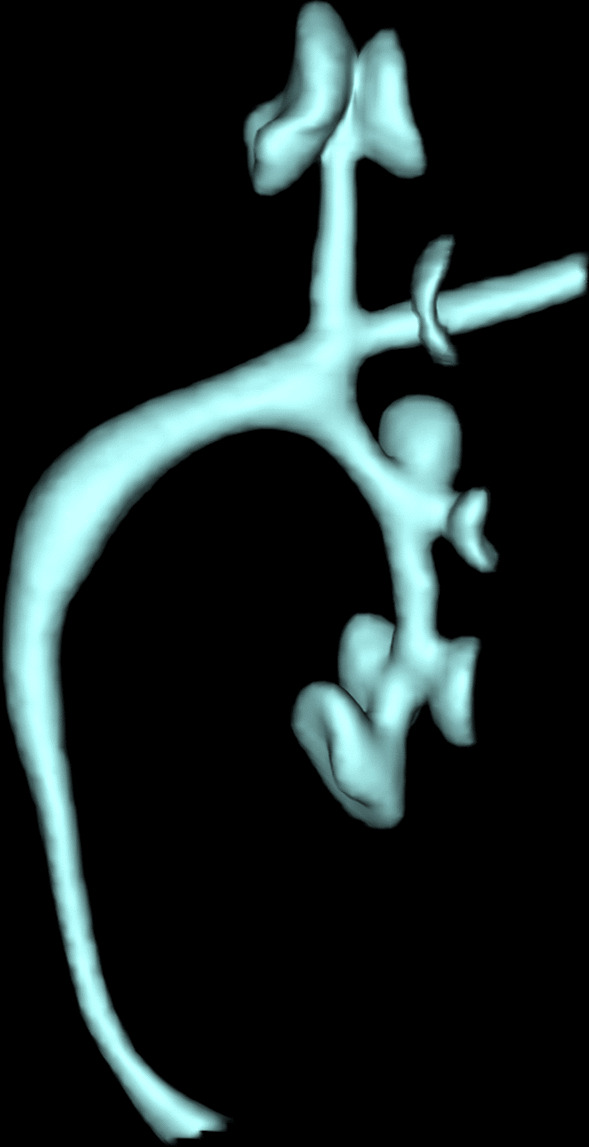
Volume data of the renal pelvis and upper ureter involved in CT detection
